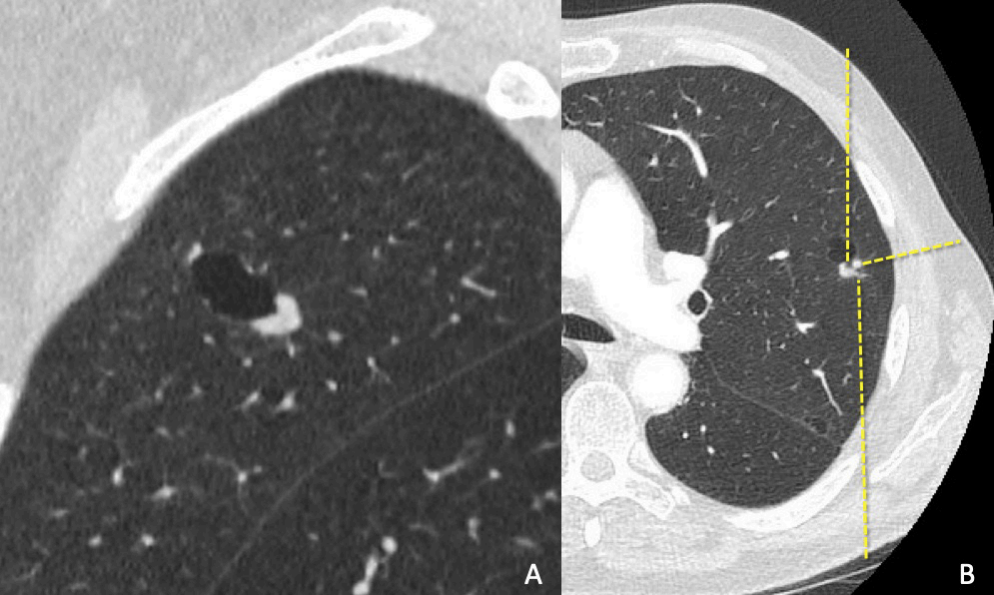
(A). A nodule with a cystic component in the left upper lobe seen in the pre-procedural CT scan. (B). Preprocedural planning with three possible marker stylet tracts (dotted lines)
